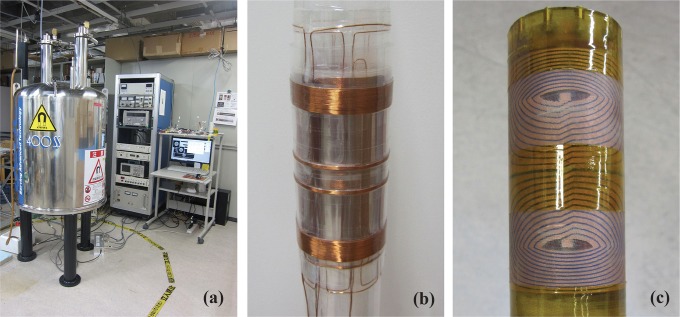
(a) Overview of the magnetic resonance imaging system using a 9.4 Tesla vertical standard bore (∼54 mm) superconducting magnet, (b) the room temperature shim coil, and (c) unshielded gradient coil. The radius of the 5-Gauss line was about 1 m.
